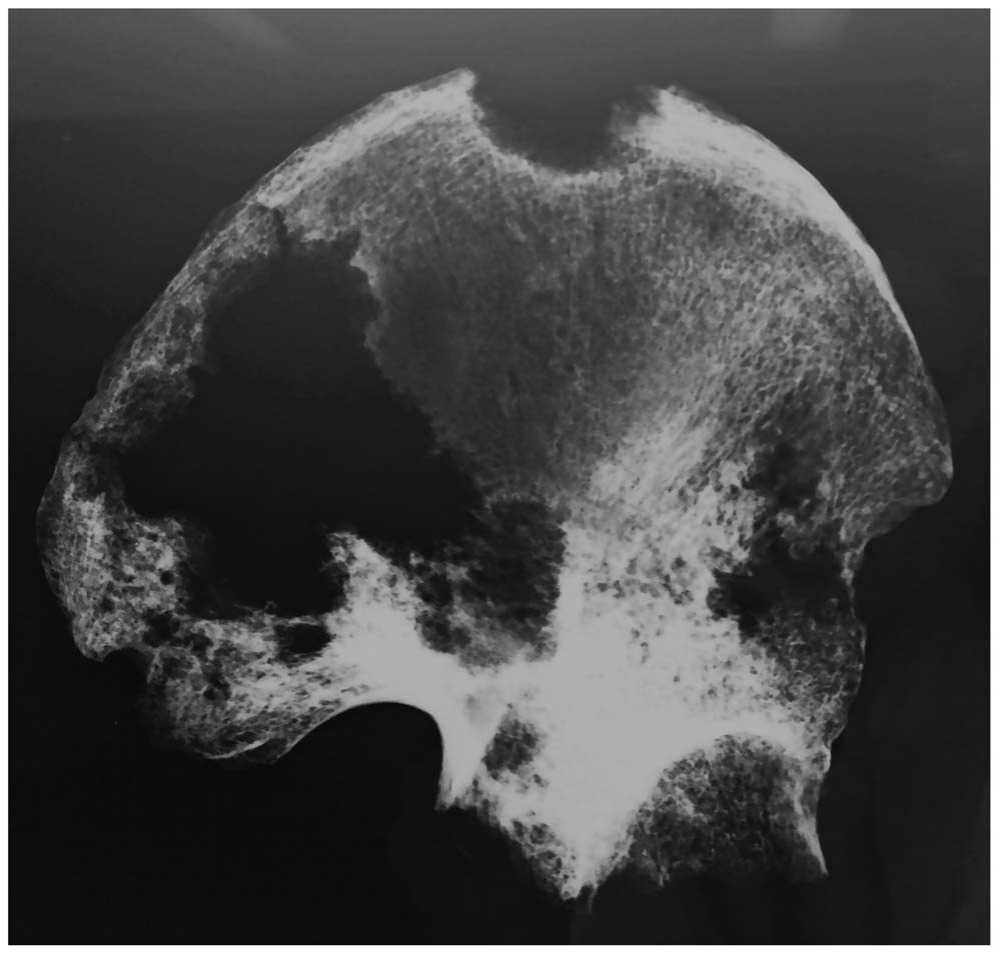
Lateral radiograph of left ilium, with osteolytic lesions indicated by areas of reduced radio-opacity.
